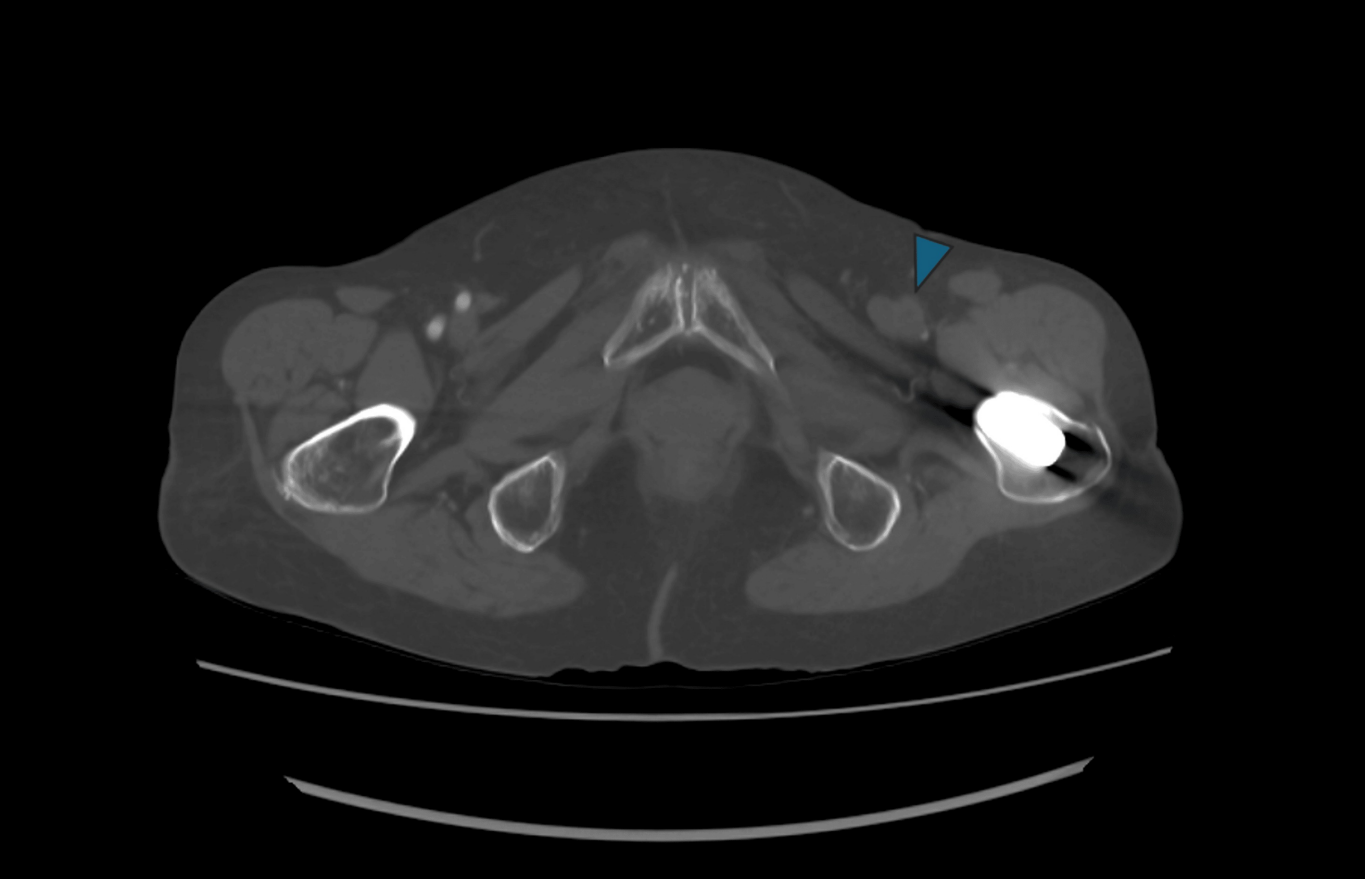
Preoperative contrast-enhanced CT angiography (axial view) Arterial wall calcification of the left femoral artery (arrowhead) was minimal. The CT attenuation value of the occluded vascular lumen was approximately 40 HU.
